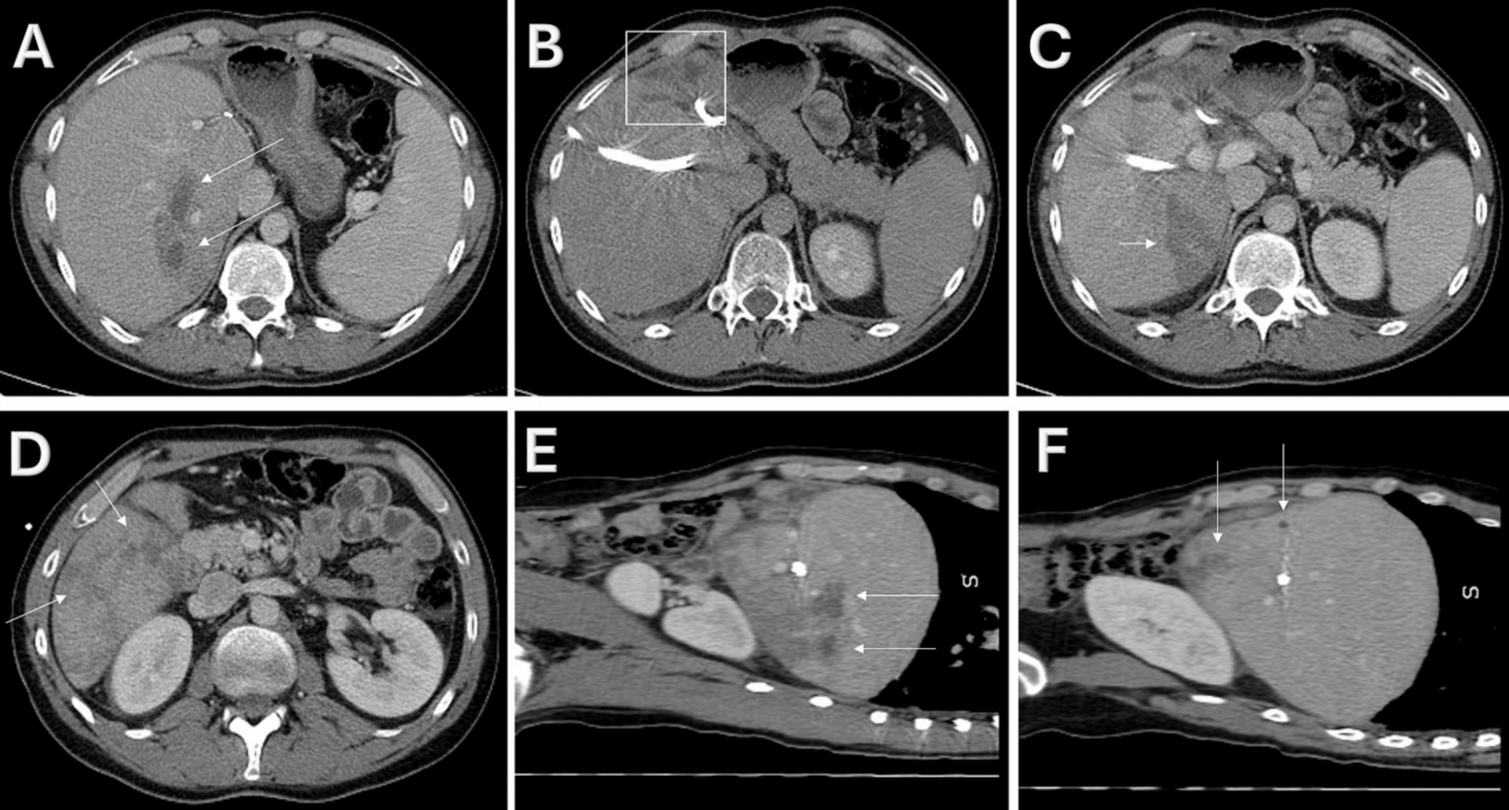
CECT scan of the abdomen (portal venous phase) showing multiple small hepatic abscesses A shows two abscesses (arrows) in segments VII and at the junction of VII and VIII; B shows subcapsular small abscesses (squares) in segments IV-B and VIII, with extension to the subhepatic area; C and D show ill-defined hypodense areas (arrows) in segments V and VI; E and F show sagittal sections revealing multiple small liver abscesses (arrows). CECT: contrast-enhanced computed tomography
